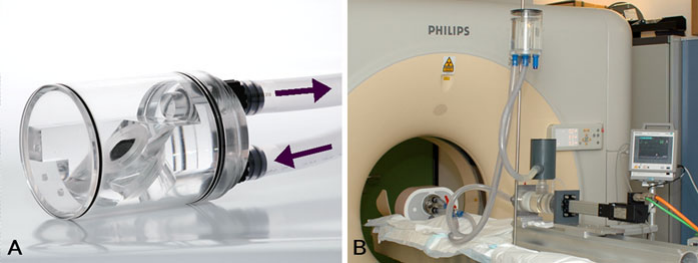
Polymethyl methacrylate (PMMA) perfusion chamber (a). Pulsatile in vitro model in 64 slice CT scanner (Brilliance 64, Philips Medical Systems, Cleveland, Ohio, USA) (b). (Reprinted with permission [9], Copyright ICR Publishers)
